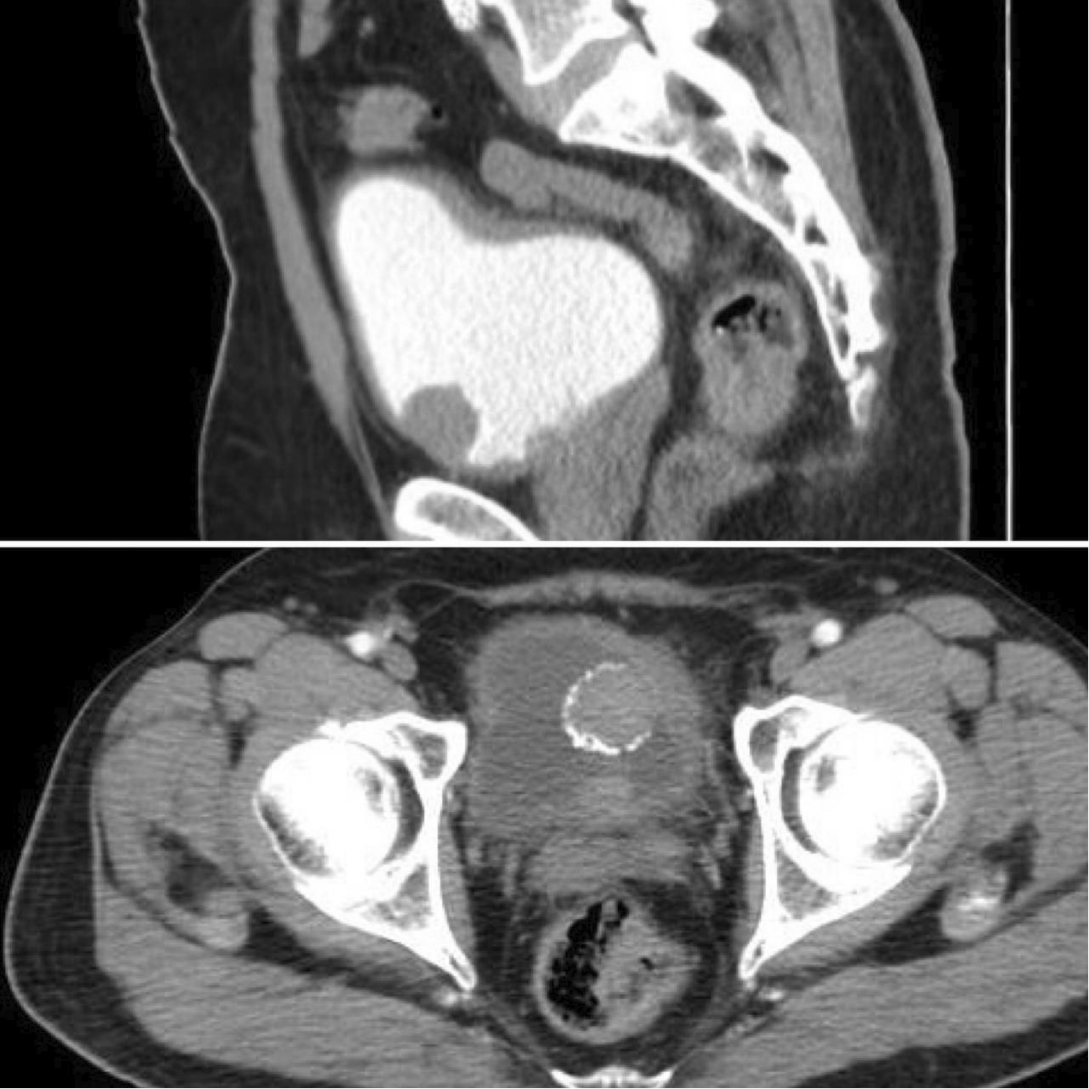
The CT scan shows the multifocal bladder tumor
